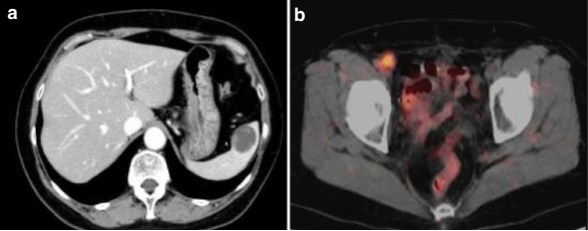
a Cystic lesion in the spleen (28 mm in diameter). b Enlarged right inguinal lymph node showing abnormal accumulation (MaxSUV = 5.0)
